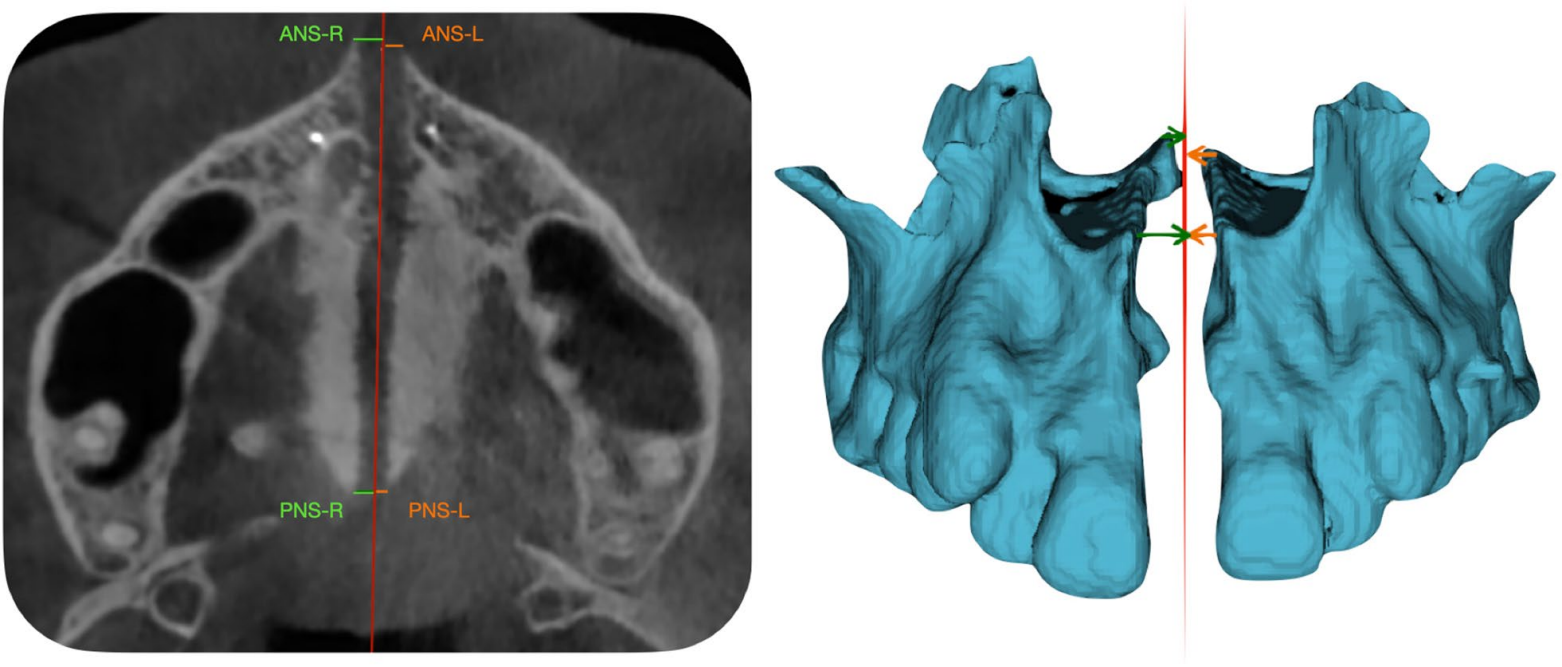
Measurements of skeletal asymmetry after maxillary expansion with MARPE. Measurements were taken on T1 CBCT (previously superimposed to T0 CBCT). Midline was taken on T0 CBCT
